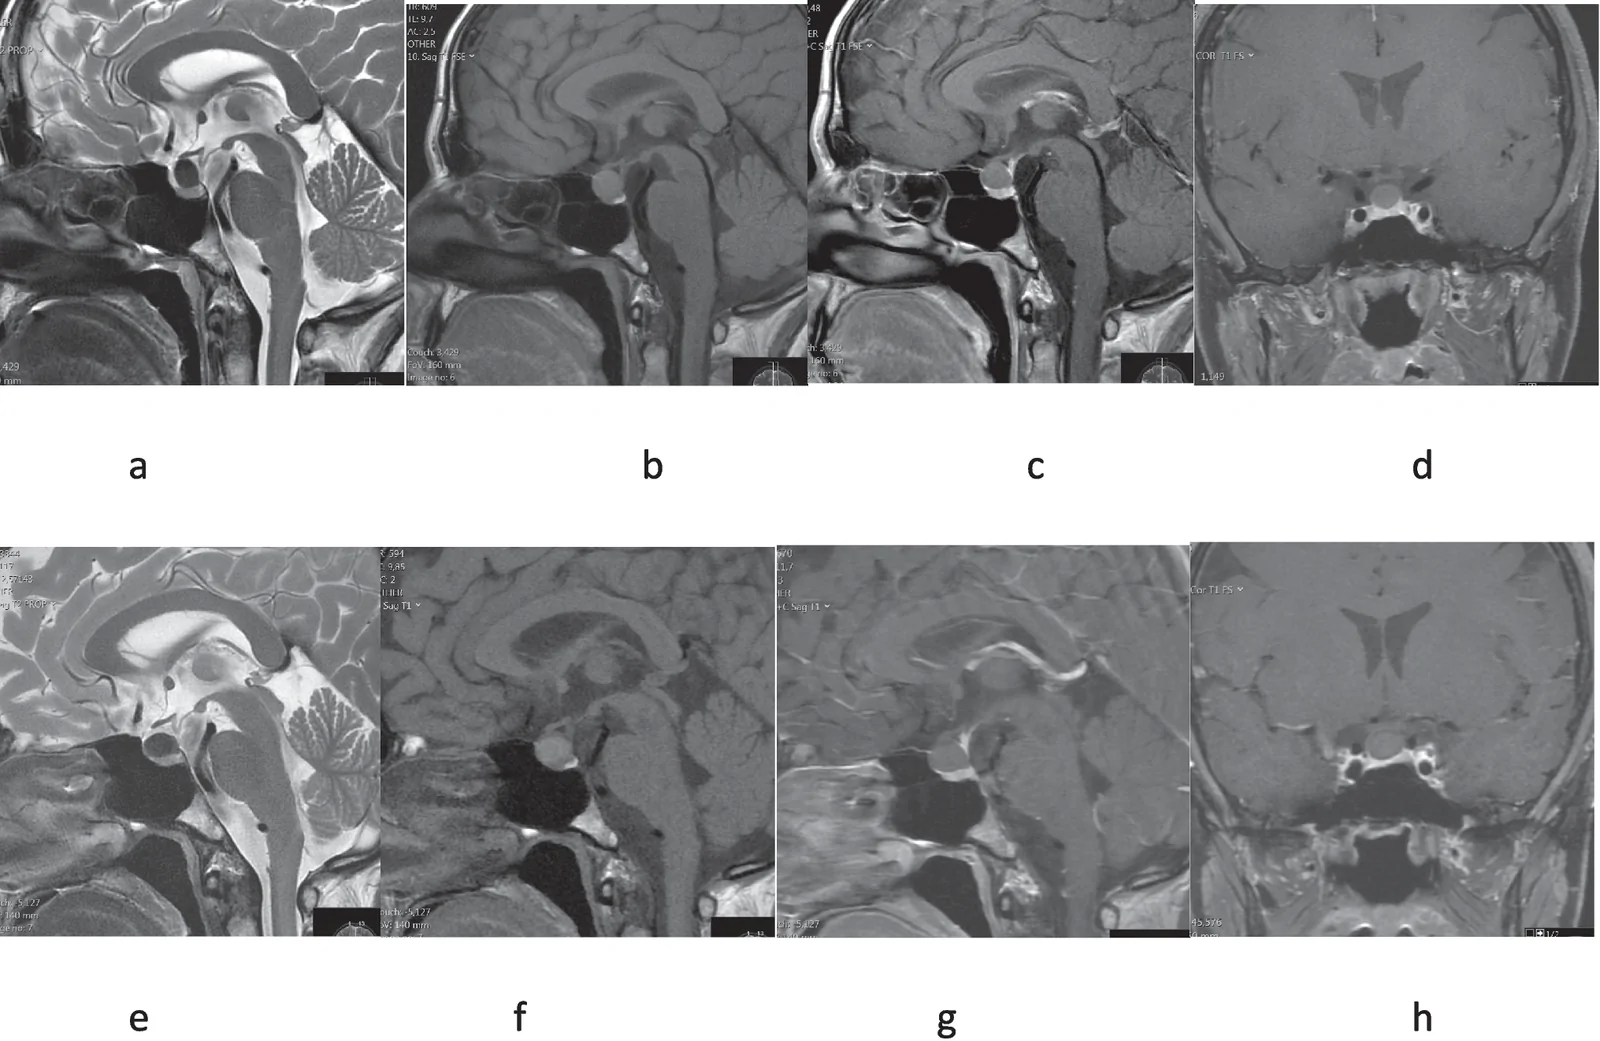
An RCC with an intracystic nodule. Magnetic resonance images show an RCC with a large nodule in the cyst that displays low signal intensity on the sagittal T2-weighted image (a) and slightly high signal intensity on the sagittal T1-weighted image (b). The cyst and intracystic nodule show no enhancement on the sagittal and coronal contrast-enhanced T1- weighted images (c, d). Magnetic resonance images taken 4 years after the initial MRI show a change in the position of the intracsytic nodule (e–h). This finding is diagnostic of an RCC
